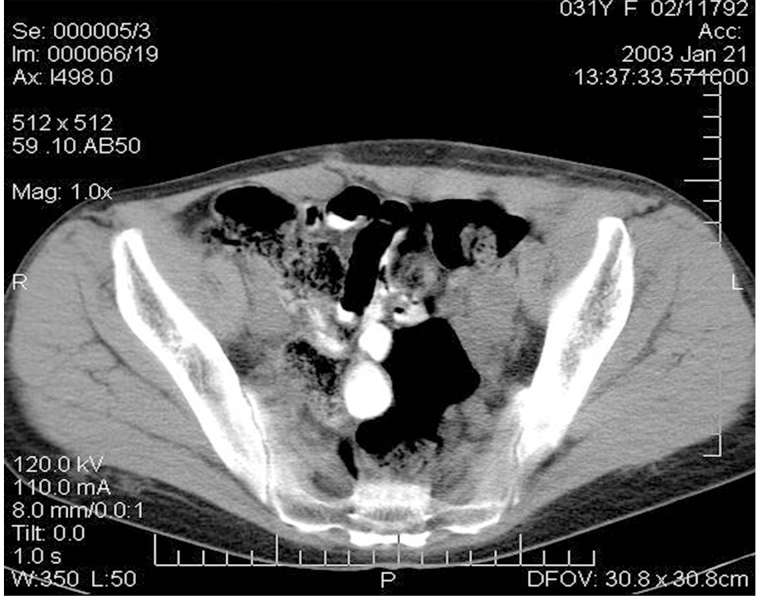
CT image of the patient’s abdomen 6 months after removal of the kidney allograft: complete regression of the lesion in the abdominal cavity
